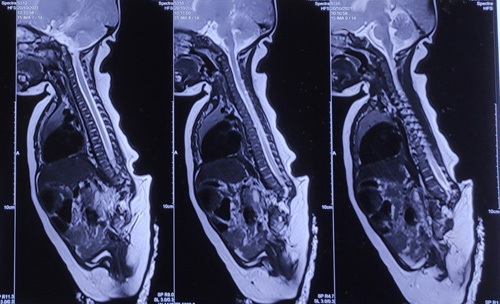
MRI spine sagittal section shows dilatation of the spinal canal of the terminal spinal cord for a distance of 2.5 cm with a maximum diameter of 2.1 mm, suggestive of syrinx. Sacrum not visualized. The iliac bones are nearly fused with each other, along with a left-sided shift of the lumbar vertebrae. The lowermost lumbar vertebra is articulating with the left iliac bone. There is evidence of a defect in the posterior arch of the L4 and L5 vertebrae without meningocele, which is suggestive of spina bifida occulta.
